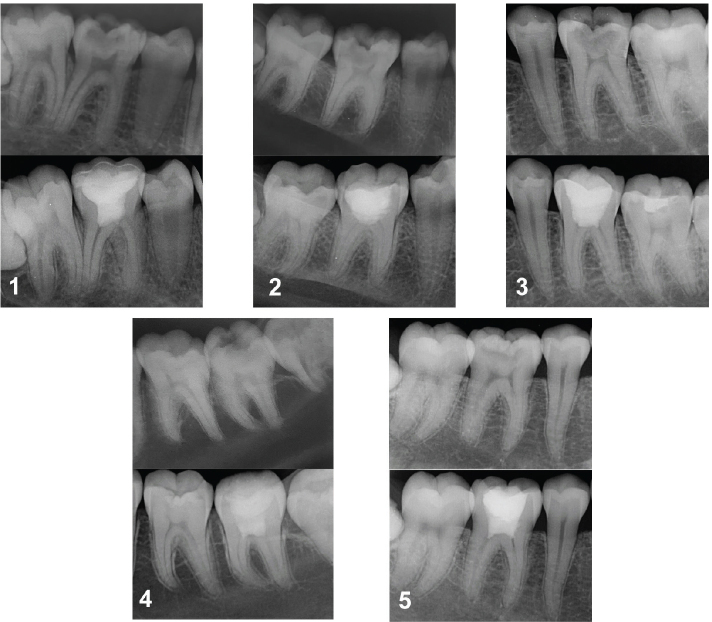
Preoperative and 1-year follow-up intra-oral periapical radiographs of cases 1–5.
